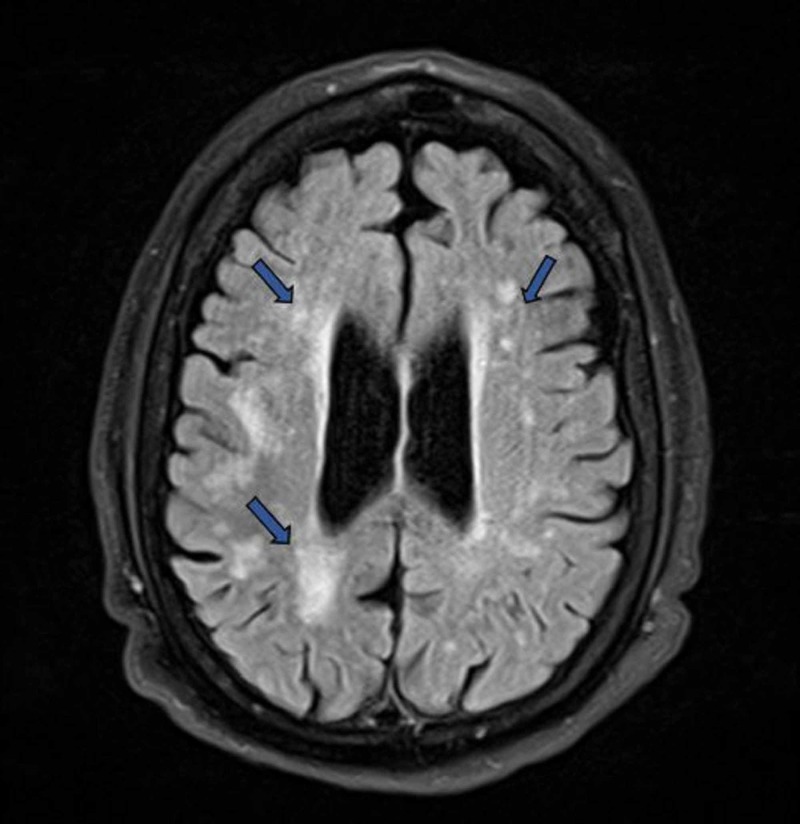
MRI of the brain showing multifocal and confluent hyperintense lesions in the periventricular white matter (blue arrows), most likely due to chronic small vessel ischemia.
